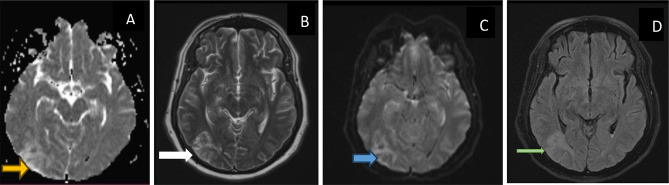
MRI Brain demonstrating sequences in axial views, including axial T2, FLAIR, DWI/ADC, and GRE/SWI. (A) Apparent Diffusion Coefficient (ADC) map shows increased signal (yellow arrow) predominantly affecting cortical and subcortical white matter of the left occipital lobe, consistent with vasogenic edema. (B) Axial T2-weighted Turbo Spin Echo (TSE) demonstrates corresponding areas of high T2 signal intensity (white arrow) within the cortical and subcortical white matter of the left occipital lobe. (C) Diffusion-weighted trace image (b = 0) reveals confluent regions of increased signal intensity (blue arrow) in the occipital cortical and subcortical white matter, supporting vasogenic rather than cytotoxic edema. (D): Axial T2-weighted FLAIR image shows extensive areas of hyperintensity (green arrow) predominantly involving the occipital & parietal cortical and subcortical white matter. This posterior-predominant distribution is characteristic of Posterior Reversible Encephalopathy Syndrome (PRES). These images, along with severe uncontrolled hypertension and acute encephalopathy, these findings are consistent with PRES with intraventricular hemorrhagic complications
